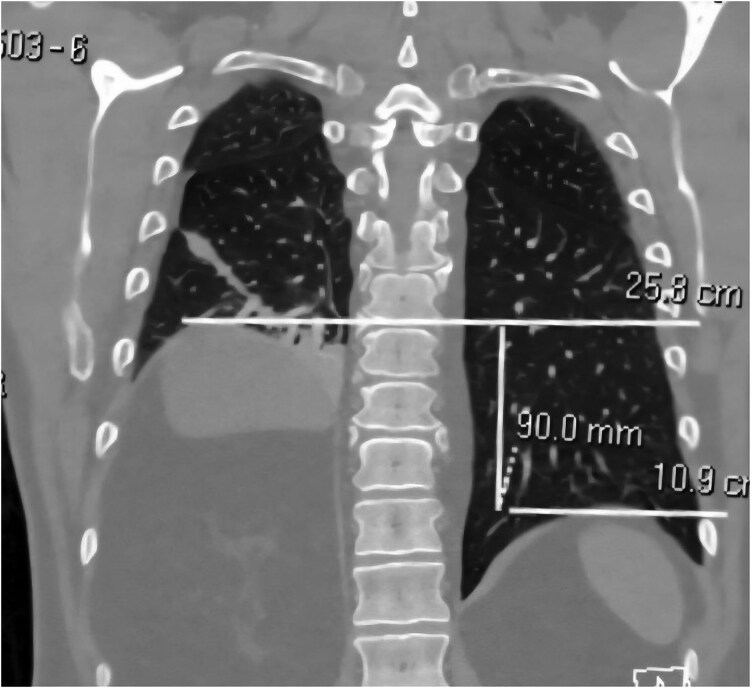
Computed tomography of the chest demonstrating elevated right-sided diaphragm ∼9 cm compared to the left-sided diaphragm.
